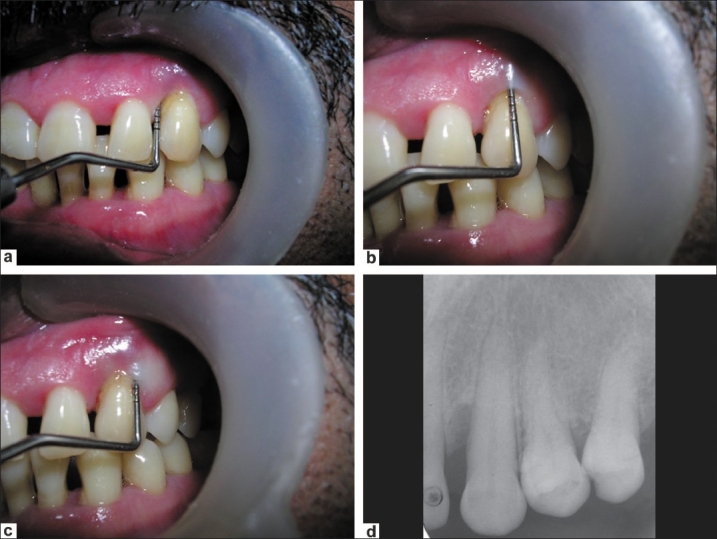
a) Periodontal probing depth was 8 mm mesially; b) Probing depth of 6 mm labially; c) Probing depth of 7.5 mm distally; d) A periapical radiograph showed a widening of the periodontal ligament space in the periapical area with an infrabony defect on the mesial aspect
